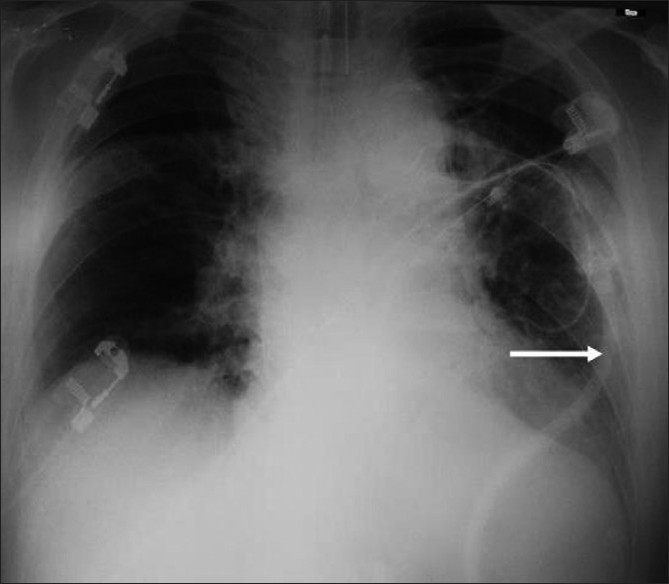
Chest X-ray of case 2 after the VPLS placement. Arrow shows the ventriculo-pleural shunt
